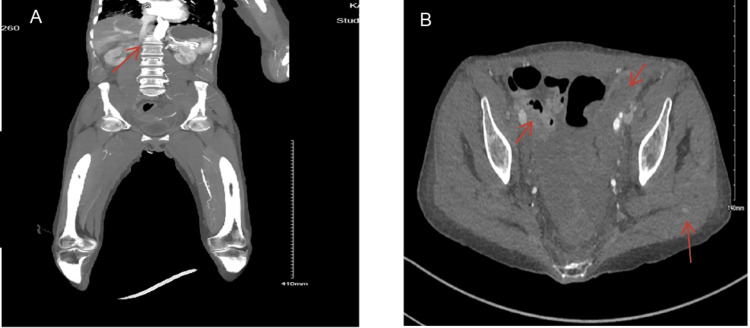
Run off CT with contrast. A: Coronal cut with almost complete occlusion in the abdominal aorta above the level of renal arteries with minimal collateral seen. B: Axial view with the bilateral iliac arteries is faintly opacified with reconstitution via the abdominal wall collaterals at the femoral level bilaterally. There is a faint hyperdensity asymmetric seen at the right glutes muscle keeping with intramuscular active arterial hematoma.
